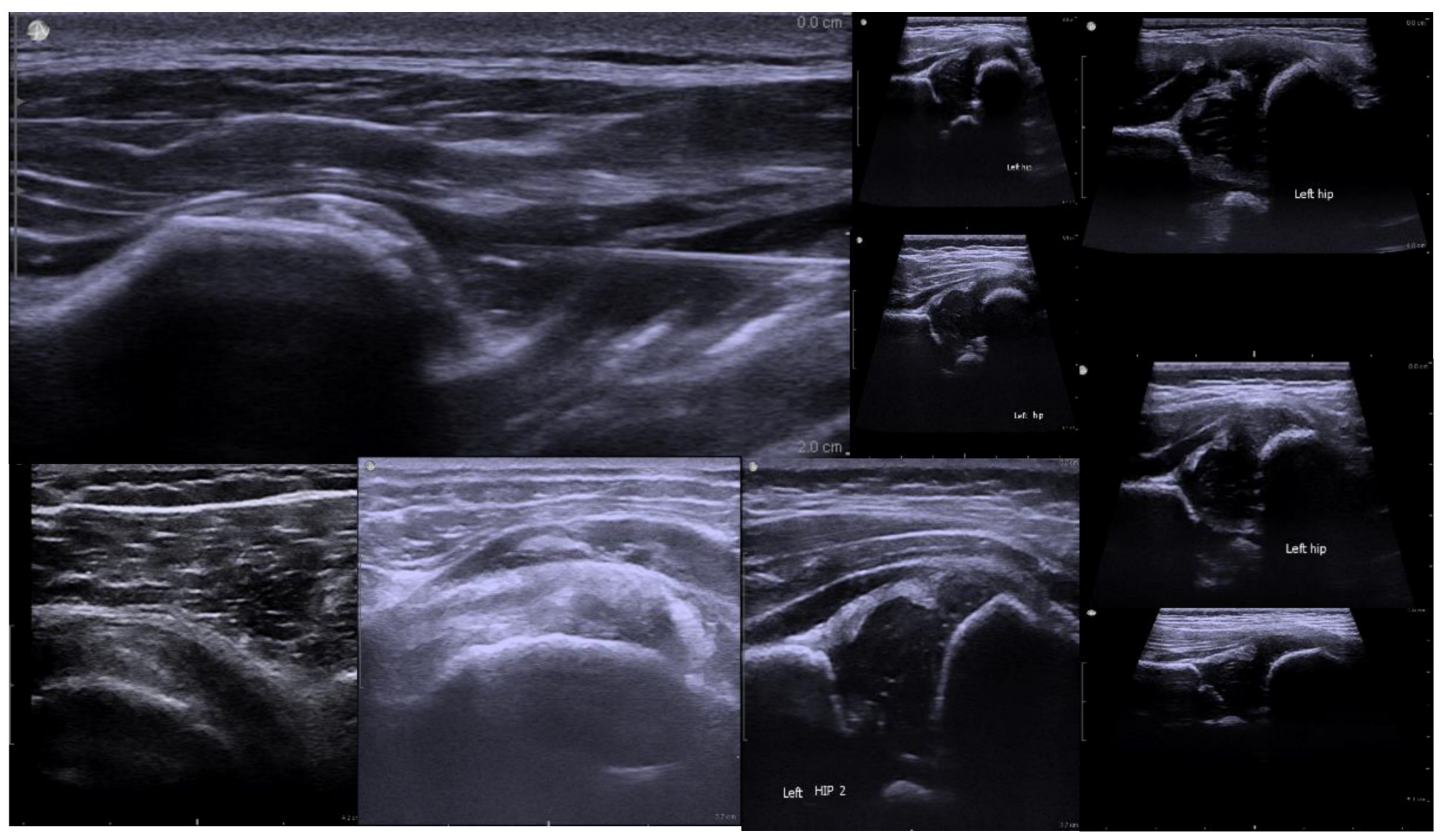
Ultrasound Image of the Hip Joint in Developmental Dysplasia of the Hip (DDH).
